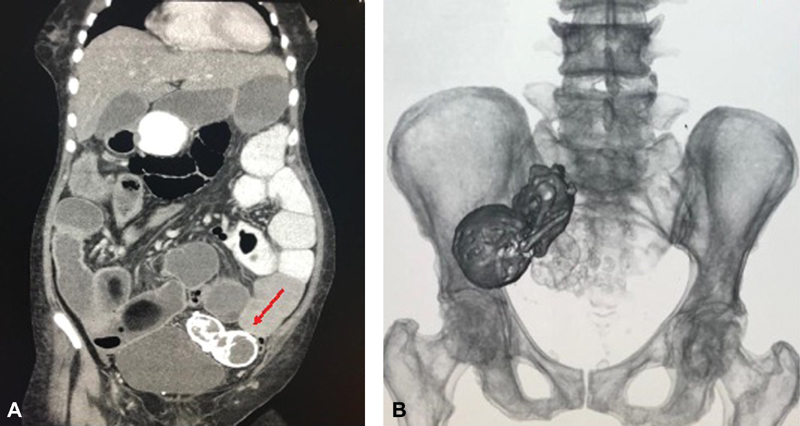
(A) Abdominal computed tomography evidencing the mummified fetus (B) Computed tomography imaging of the abdomen with 3D reconstruction.
